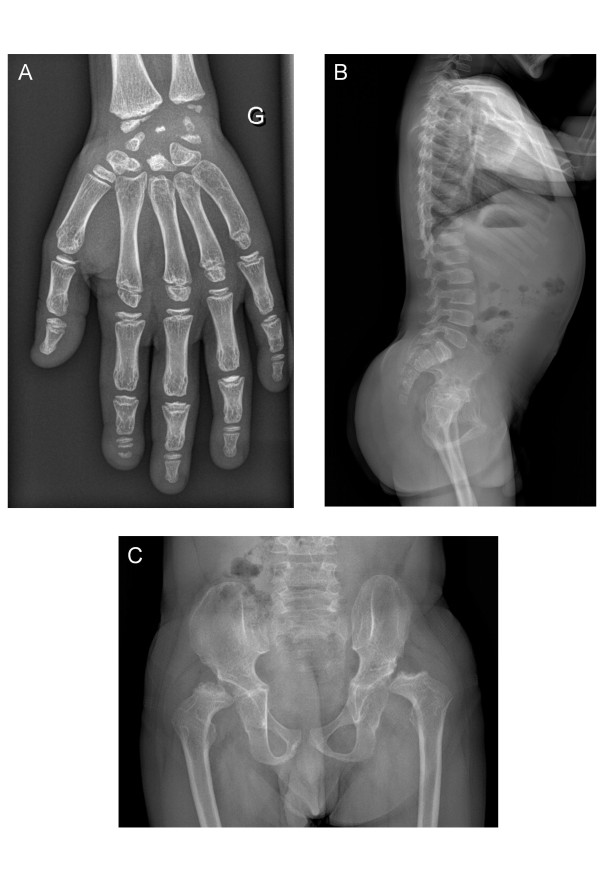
Radiographs of a WRS patient (age 13 years). A. Hand: the carpal bones are small and irregular with dysplastic distal radial and ulnar epiphyses; several phalanges are dysplastic with abnormal metaphyseal cupping at the proximal ends. B. Spine: dorsal region of spine shows flattening of the vertebral bodies with defects at the anterior edges. C. Pelvis: the acetabular roofs are hypoplastic with dysplastic capital femoral epiphyses.
